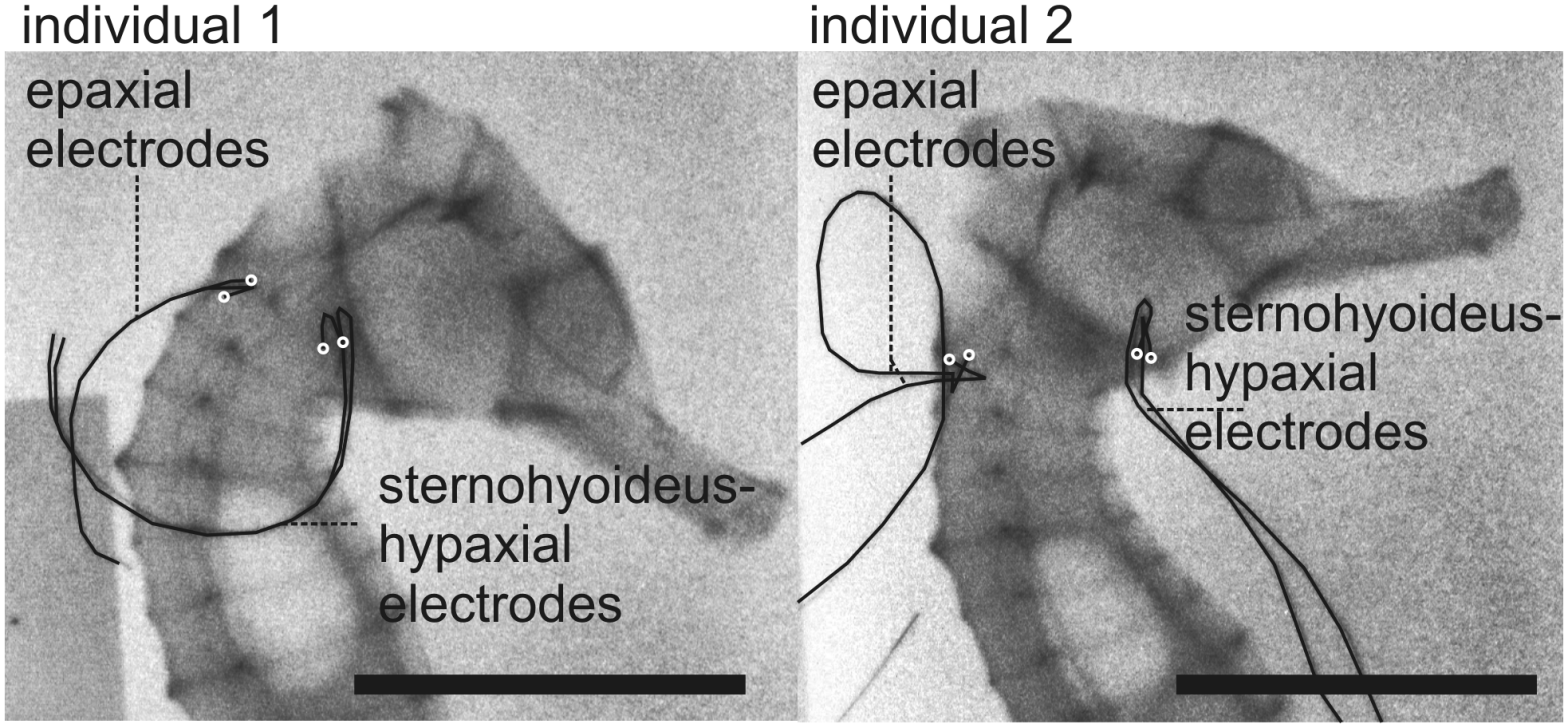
X-ray images showing the placement of the electrodes for both individuals in the electromyography analysis. The uncoated electrode tips are indicated by white circles. Scale bars, 10 mm.
